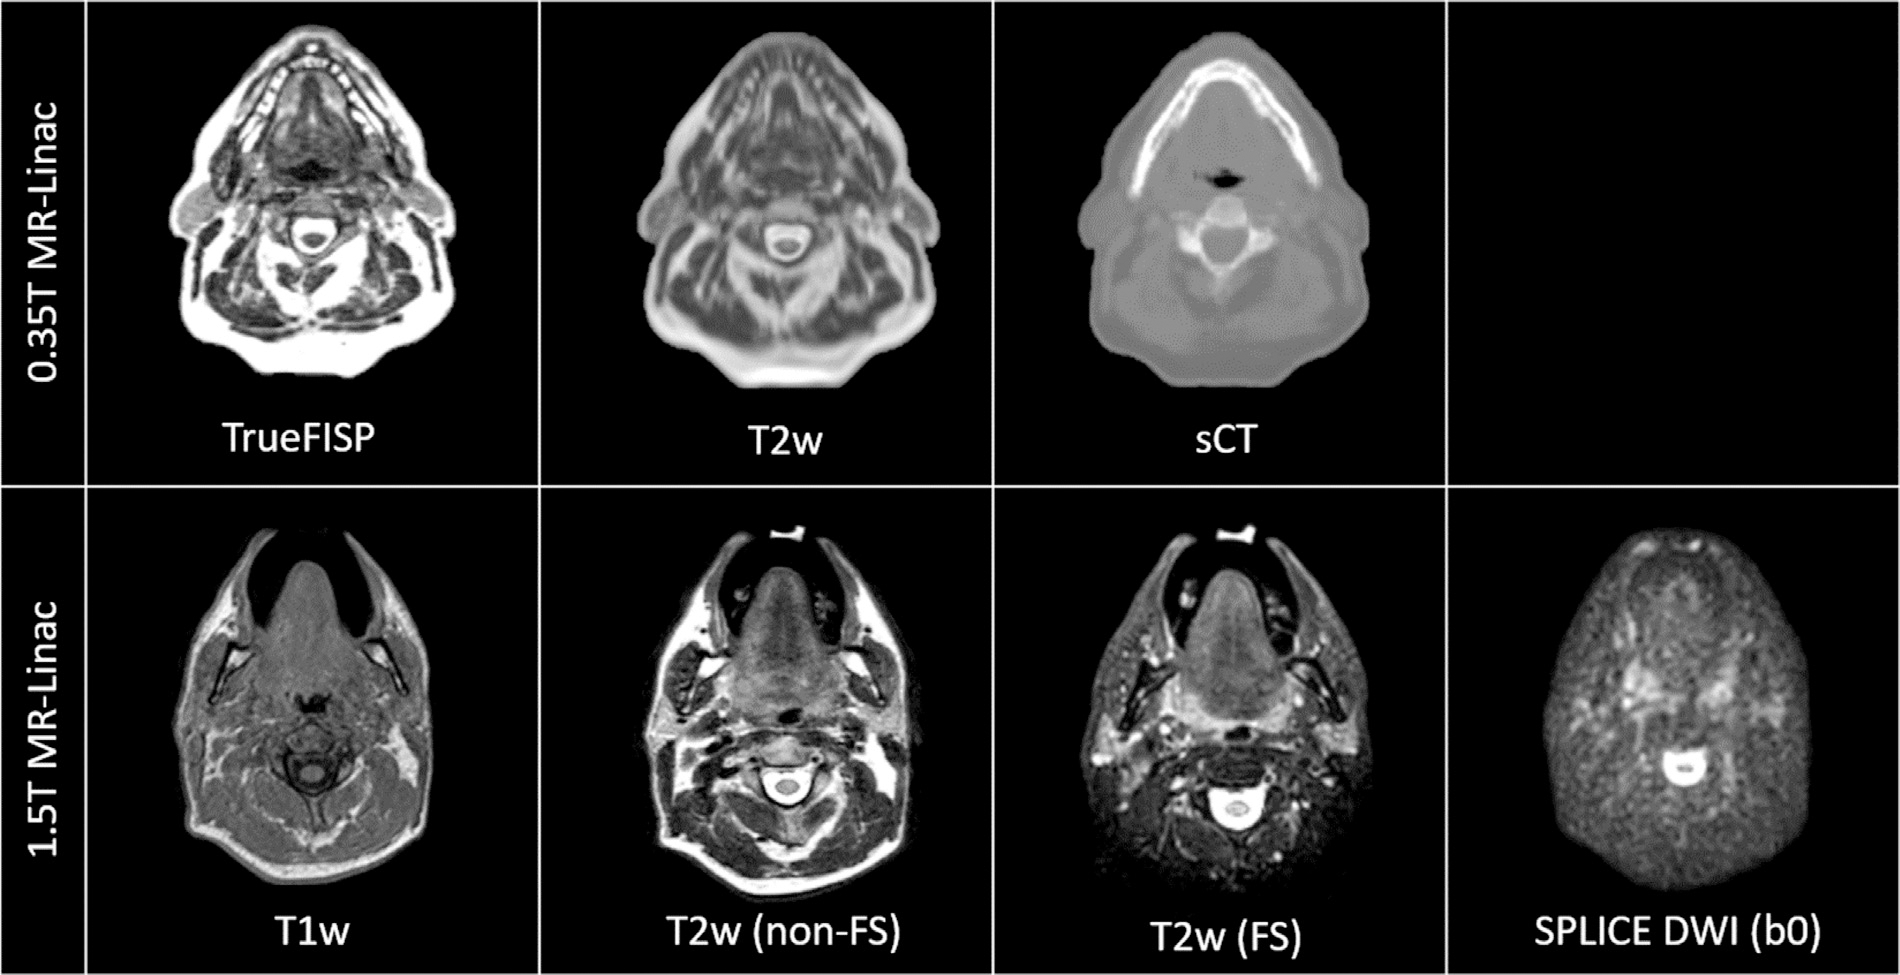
Selected sequences acquired on the 0.35T and 1.5T MR-linac systems. Abbreviations: TrueFISP, true fast imaging with steady-state free free precession; T1w, T1-weighted; T2w, T2-weighted; FS, fat-suppressed; sCT: synthetic CT; SPLICE, split acquisition of fast spin echo signal for diffusion imaging; DWI, diffusion-weighted imaging. (Color version of figure is available online.)
